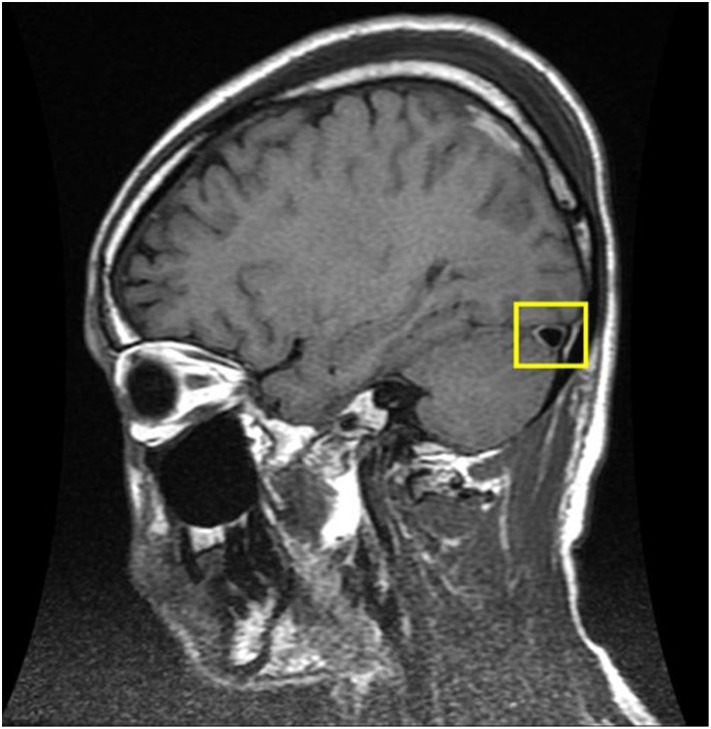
Typical MRI manifestation of the venous distension sign. T1-weighted MRI through the midportion of the dominant transverse sinus shows the venous distension sign (box).
